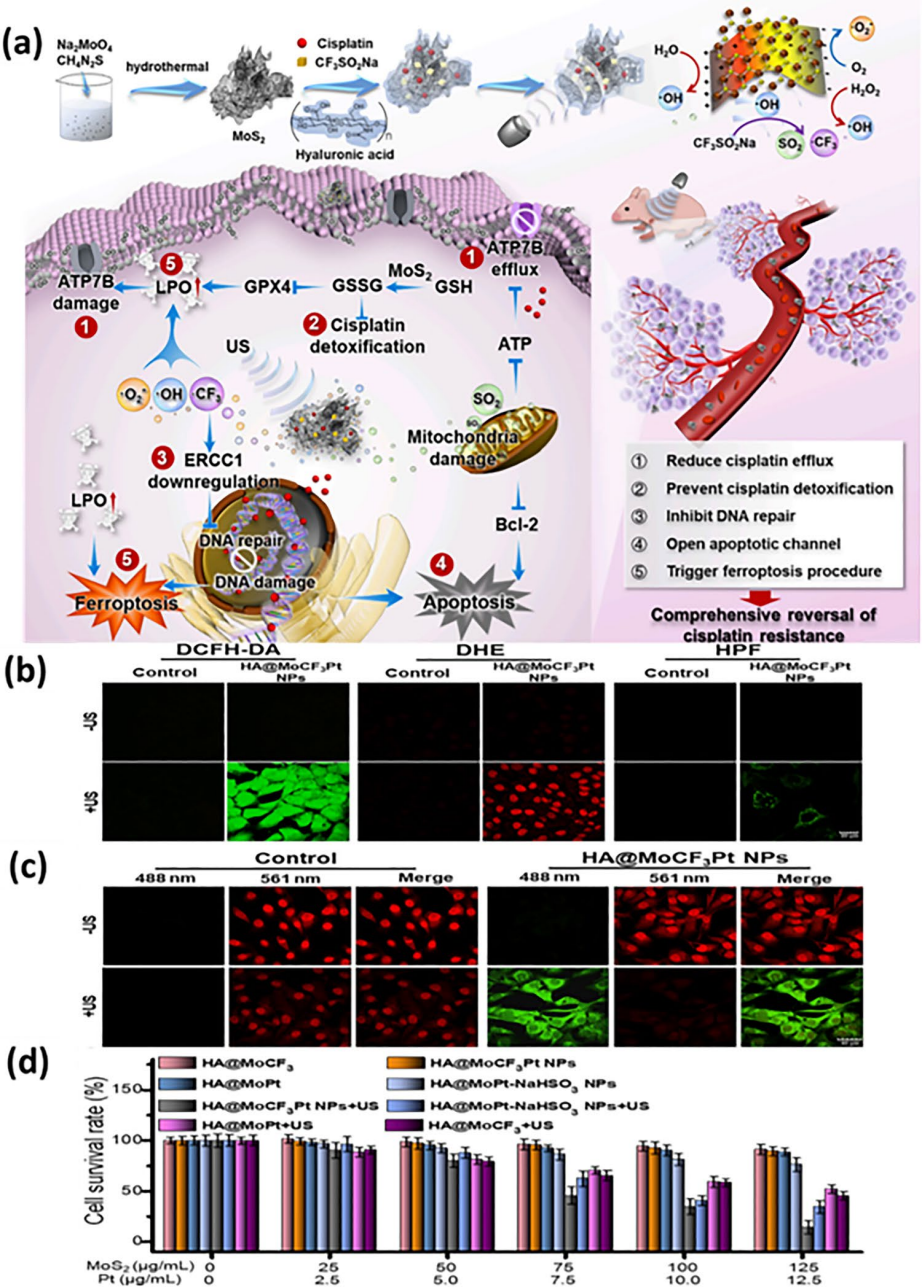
a Synthesis of SO2, •CF3 and •OH bearing nanoflower and its inhibiting mechanism for cisplatin resistance cancer under ultrasonic radiation, b ROS suppressing effect of nanoflower detected by 2,7-dichlorodihydrofluorescein diacetate (DCFH-DA), dihydroethidium (DHE) and hydroxyphenyl fluorescein (HPH), c determination of SO_2_ production capacity coumarin–hemicyanine dye, d cell cytotoxicity study of different nanopreparations under US irradiation. Reproduced with permission from Ref. [193]
